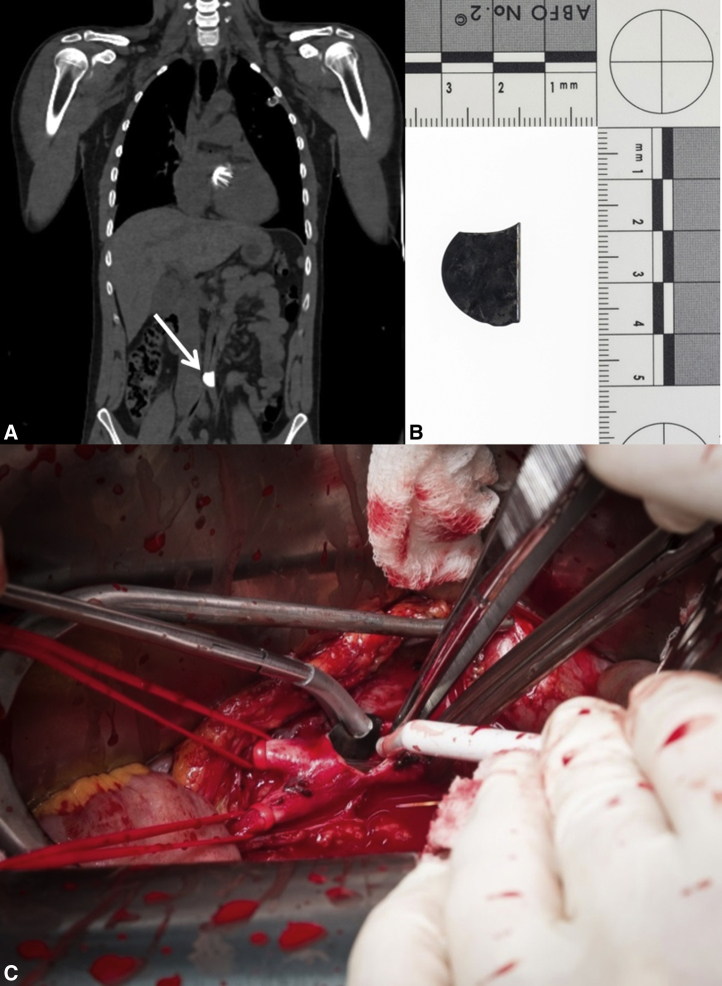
A, Postoperative computed tomography scan without contrast showing the location of the embolized leaflet in the abdominal aorta (*white arrow*). Three quarters of the missing valve lid (B) was removed from the abdominal aorta via laparotomy (C). The remaining one-quarter was not accounted for but was suspected to lay distal to the knee, the only area not imaged.
